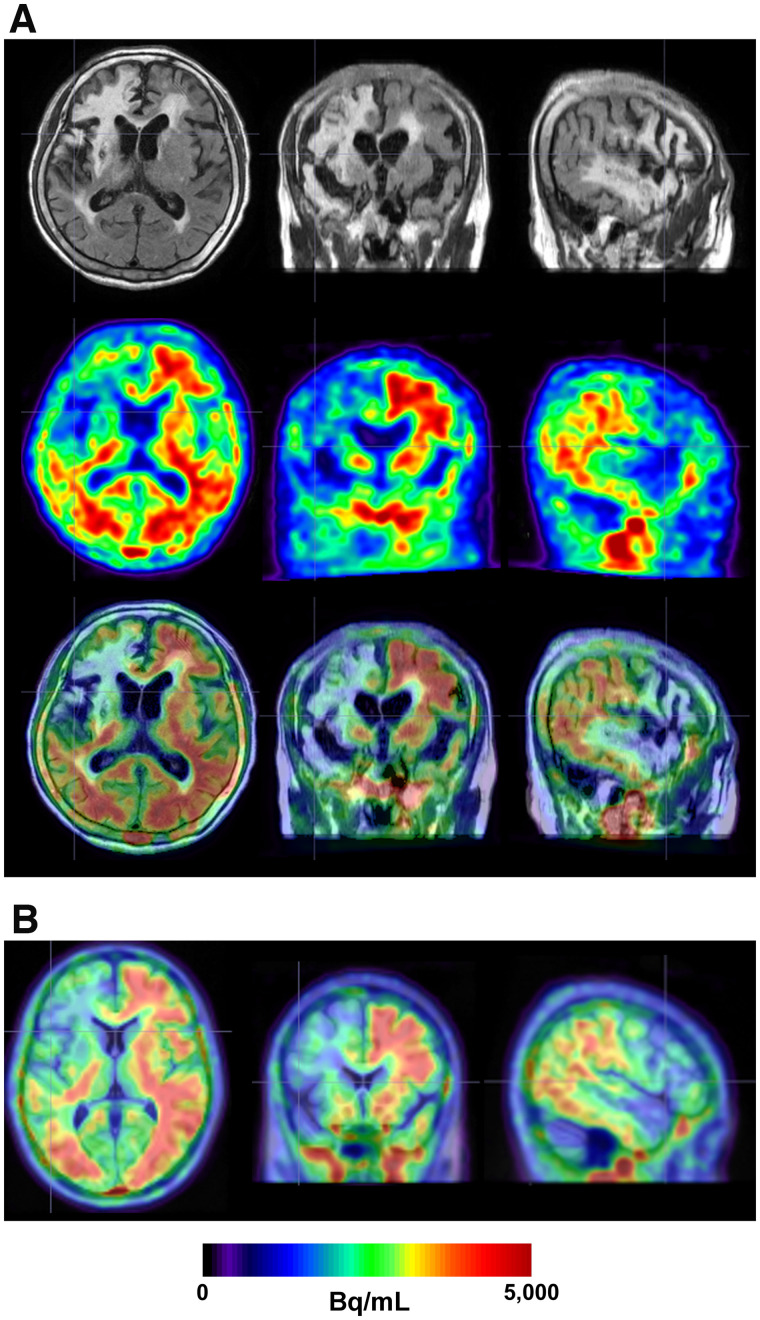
SN result of patient with chronic stroke lesion using proposed method. (A) Patient’s original FLAIR MRI (top), ^18^F-florbetaben (middle), and PET/MRI fusion (bottom). (B) SN PET overlaid on standard T1 MRI template.
